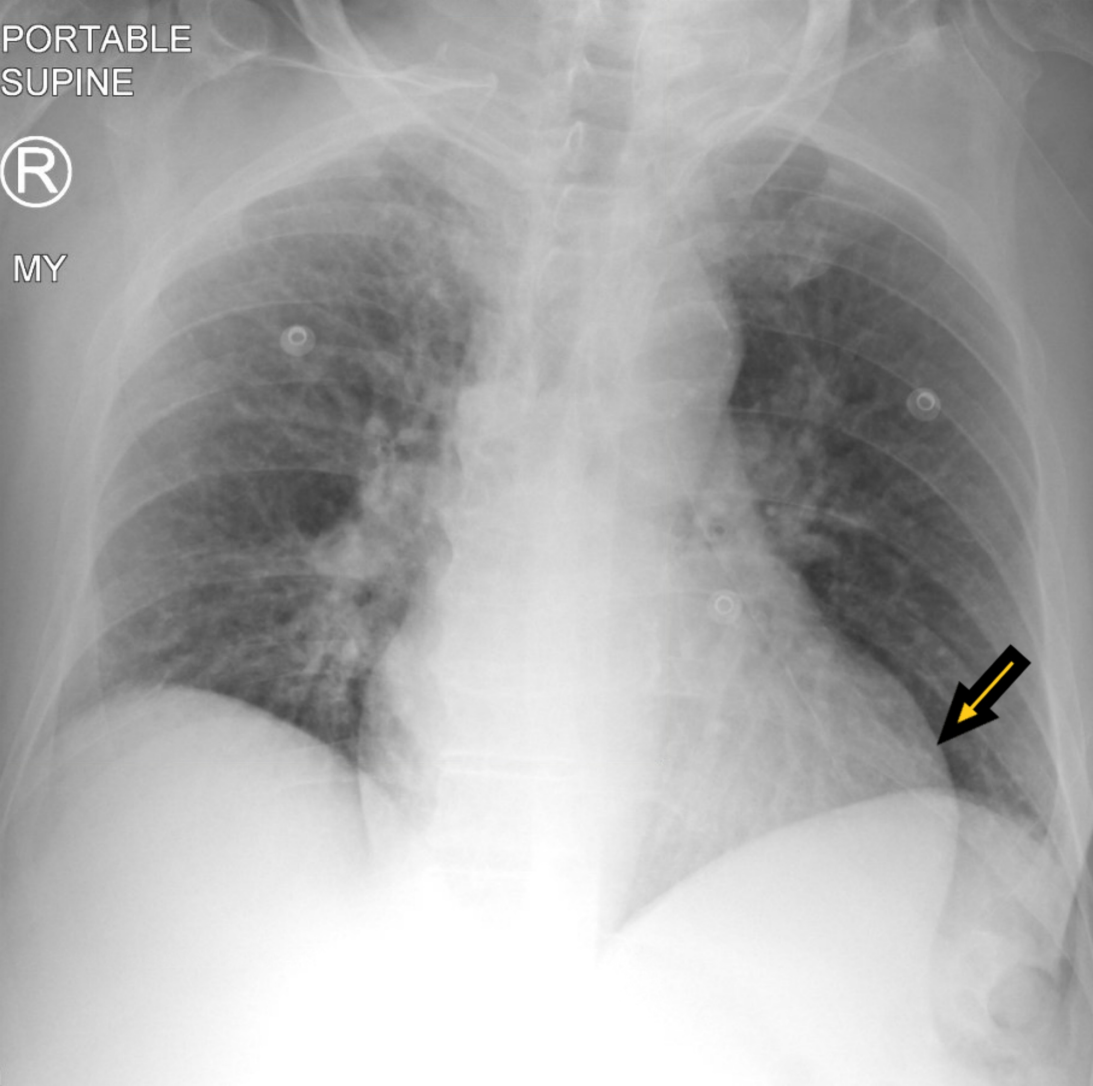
Chest X-ray showing cardiomegaly with no acute lung infiltrates.
